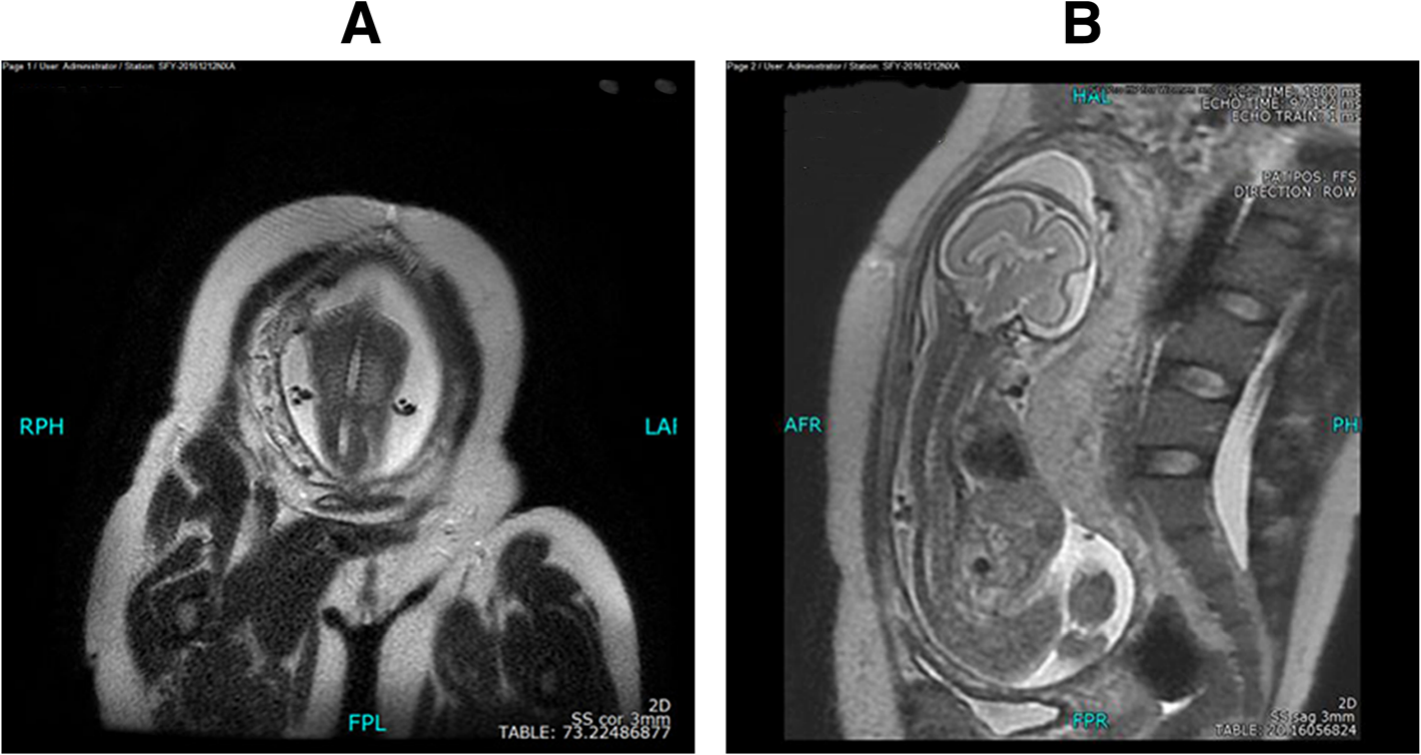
MRI features in our patient. a & b. MRI showed the disappeared normal curvature of the fetal spine, partial depression of lower thoracic segment, and abnormal and narrow down of the spinal canal
